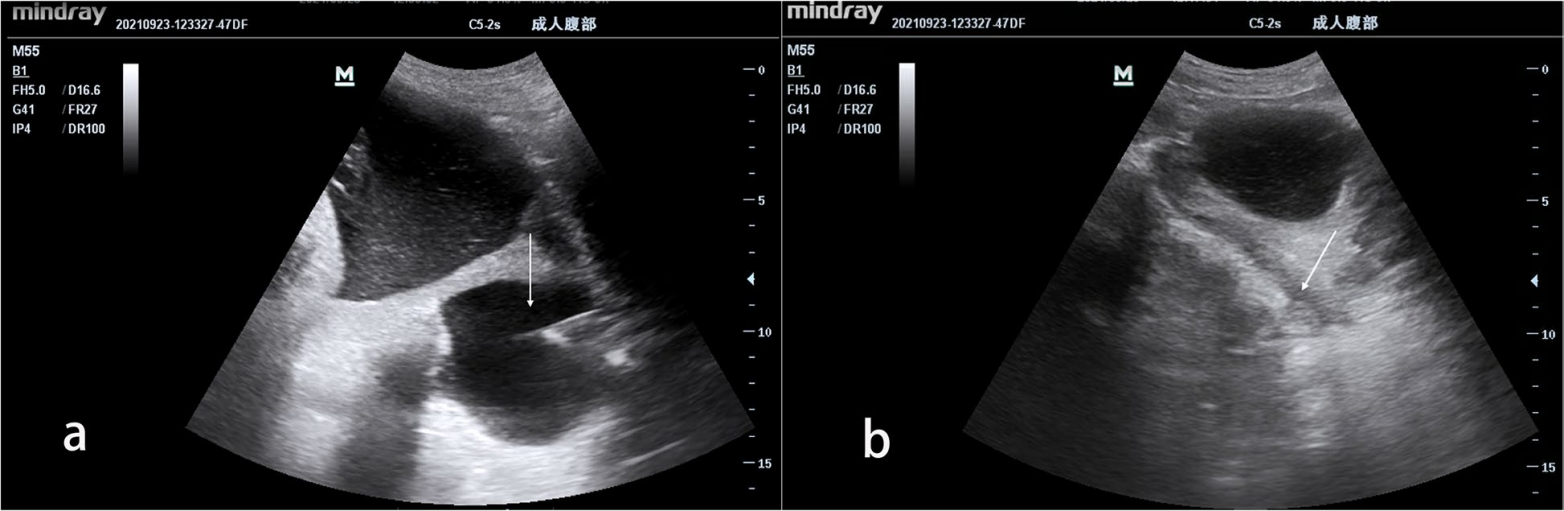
a Sonography at 37 weeks of gestation. It shows draining of the liquid of the cyst through the posterior fornix under the guidance of ultrasound (arrow). b Sonography at 37 weeks of gestation. After draining of the liquid of the pelvic hydatid cyst through the posterior fornix under the guidance of ultrasound, the cyst disappeared (arrow)
